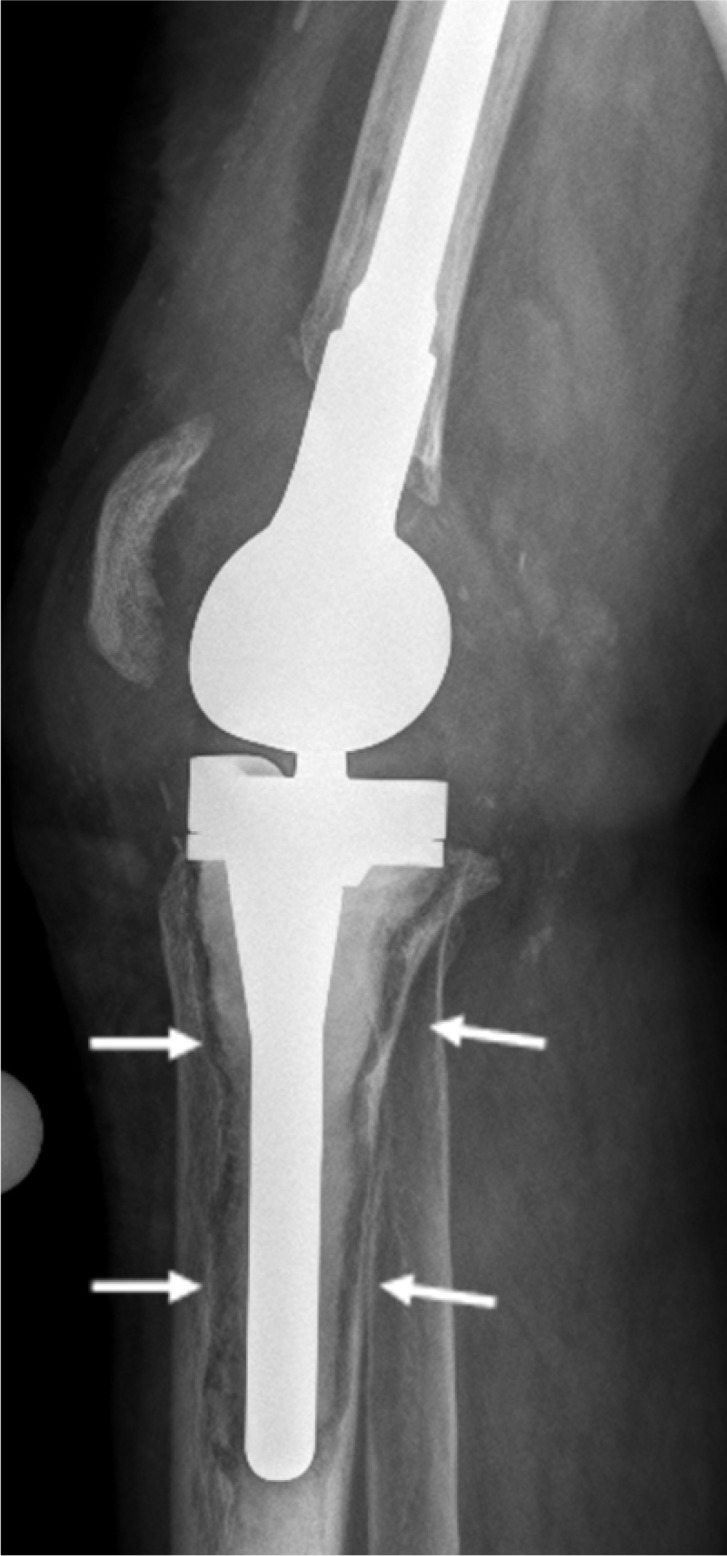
X-ray of a patient suffering from infectious implant loosening of a total knee replacement. Radiolucent lines can be seen particularly around the tibial implant component, indicative of loosening (white arrows).
